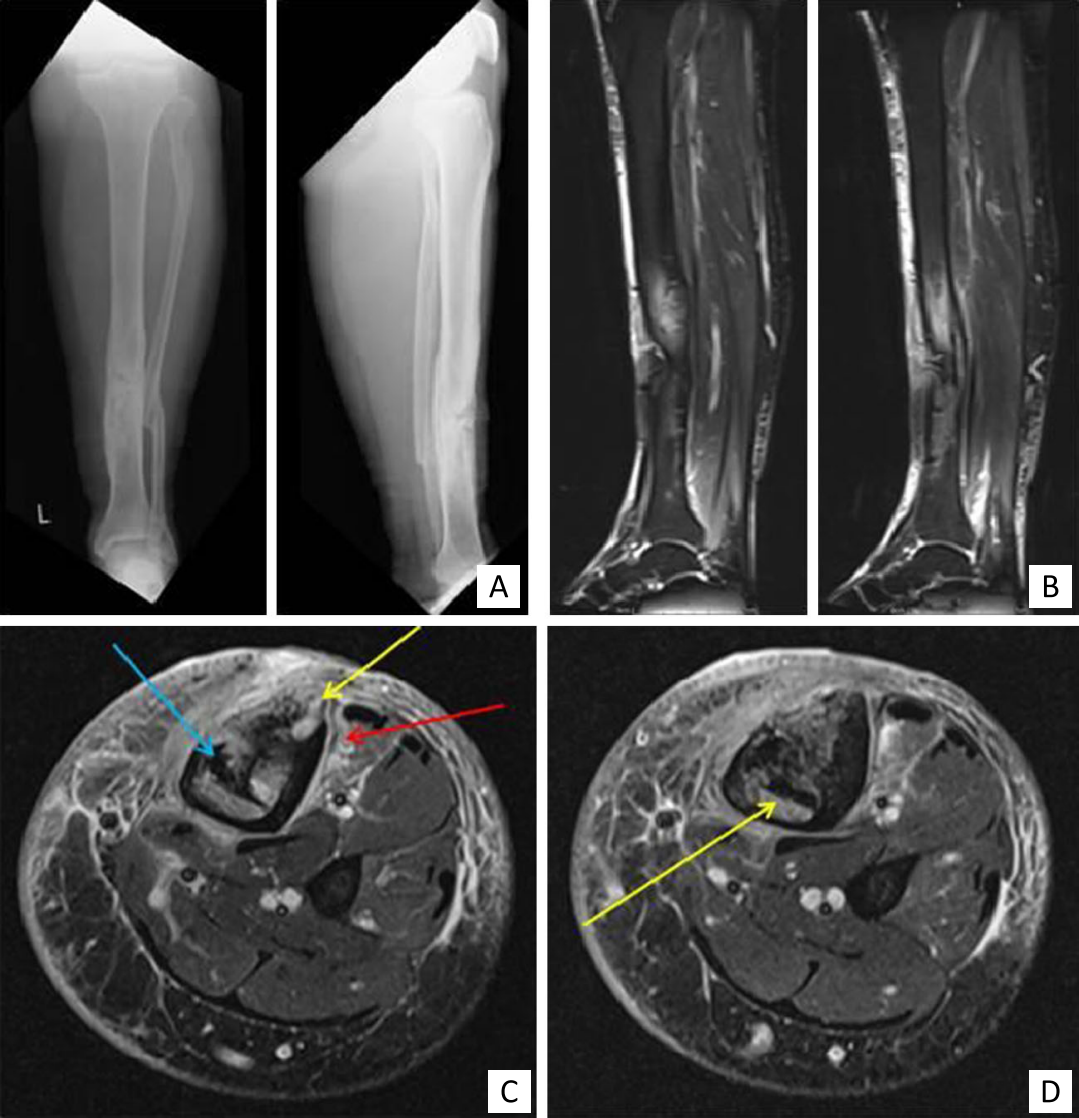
Clinical example of MRI. A 54-year-old man with a history of an open fracture treated with a plate many years ago. The fracture healed slowly and then the plate was removed because of continued skin breakdown over the front of the tibia. a Frontal and lateral radiograph demonstrating sclerosis and chronic periosteal reaction around the previous fracture site. b Sagittal fat-suppressed images of the calf demonstrating bone and soft tissue oedema. c & d Axial fat-suppressed images demonstrating sequestra (blue arrow), cortical abscesses (yellow arrows) and periostitis and soft tissue oedema (red arrow)
